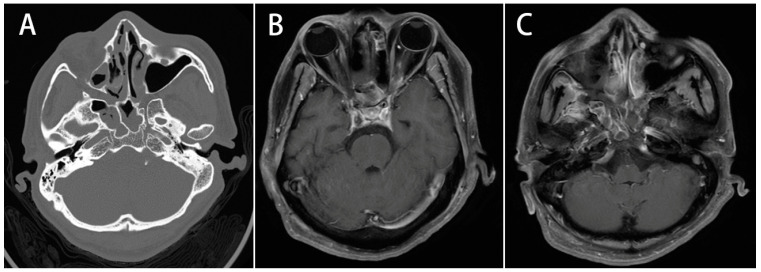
Image study of case 3. A 44-year-old man with a history of poorly controlled diabetes presented with eyelid swelling, blindness, alert mental status, mydriasis, fever, and headache. (A): Axial contrast-enhanced CT scan. CT scan revealed rhinosinusitis over bilateral sphenoid sinuses, complicated with subperiosteal empyema, bony wall erosion, and right orbital infection. (B,C): Axial T1-weighted MRI images. MRI showed bilateral bulging of the cavernous sinus and signal enhancement over cavernous sinus and skull base under T1-weighted images.
